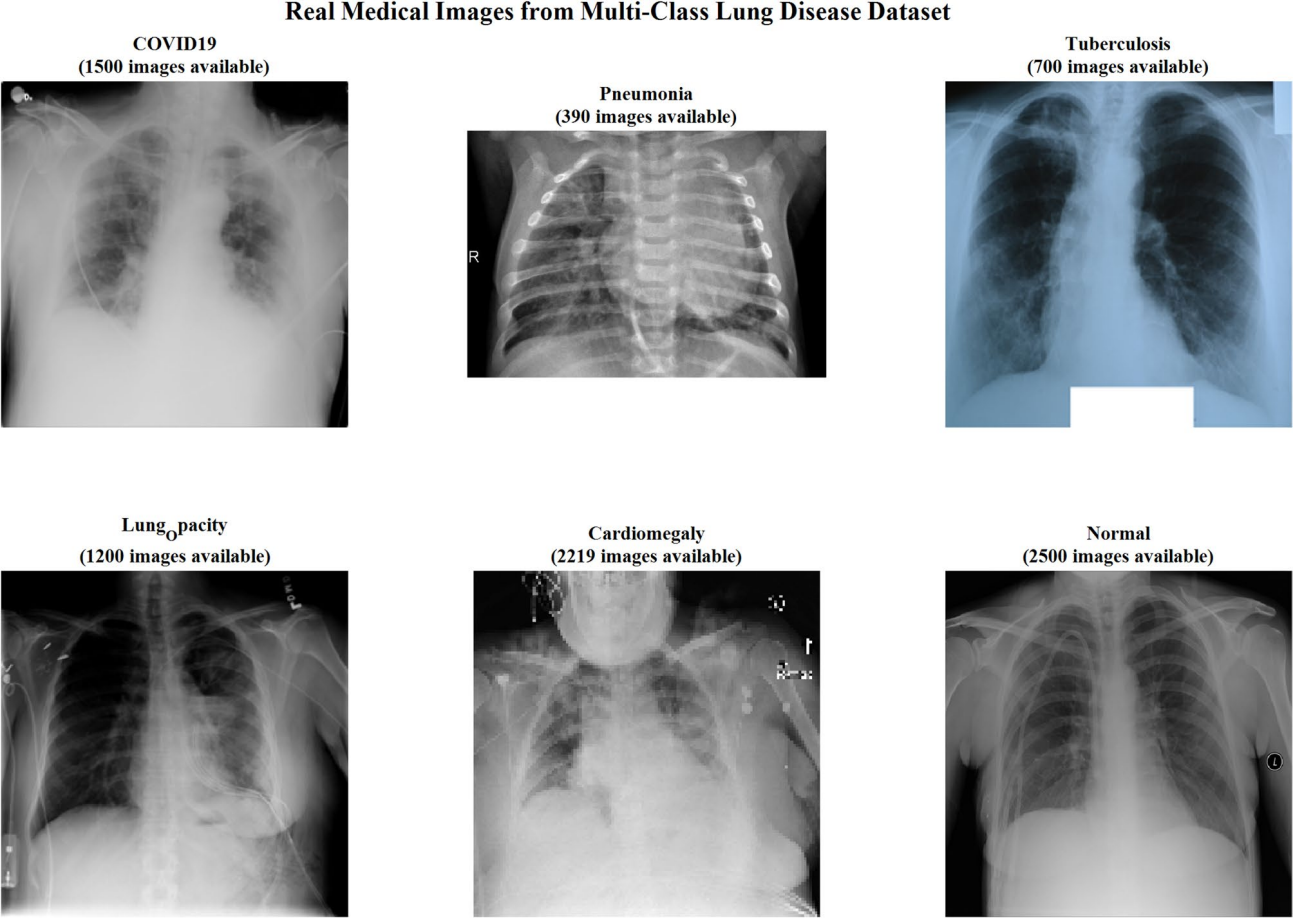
Representative chest X-ray Images from multi-class lung disease dataset with class distribution.
